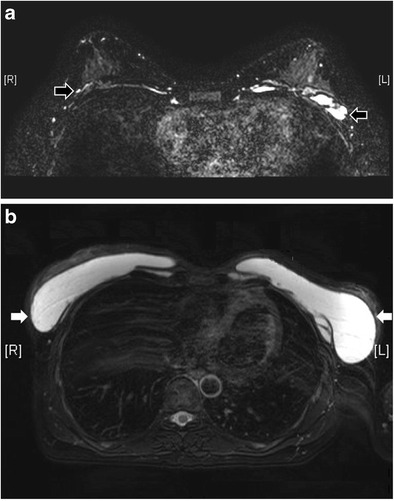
PAAG at anterior abdominal wall. Axial T2-weighted fat-suppressed image show there is minimal PAAG in bilateral retroglandular spaces (black arrows) (a). Instead, the main collections are found over anterior abdominal wall (white arrows) (b)
